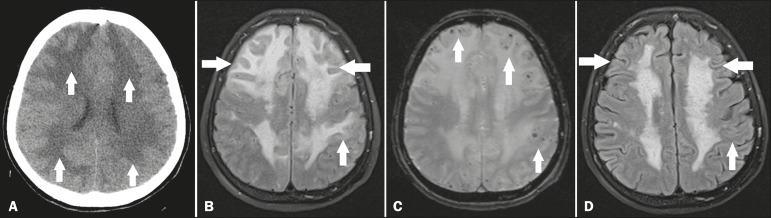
A: Noncontrast axial CT showing marked, diffuse bilateral
